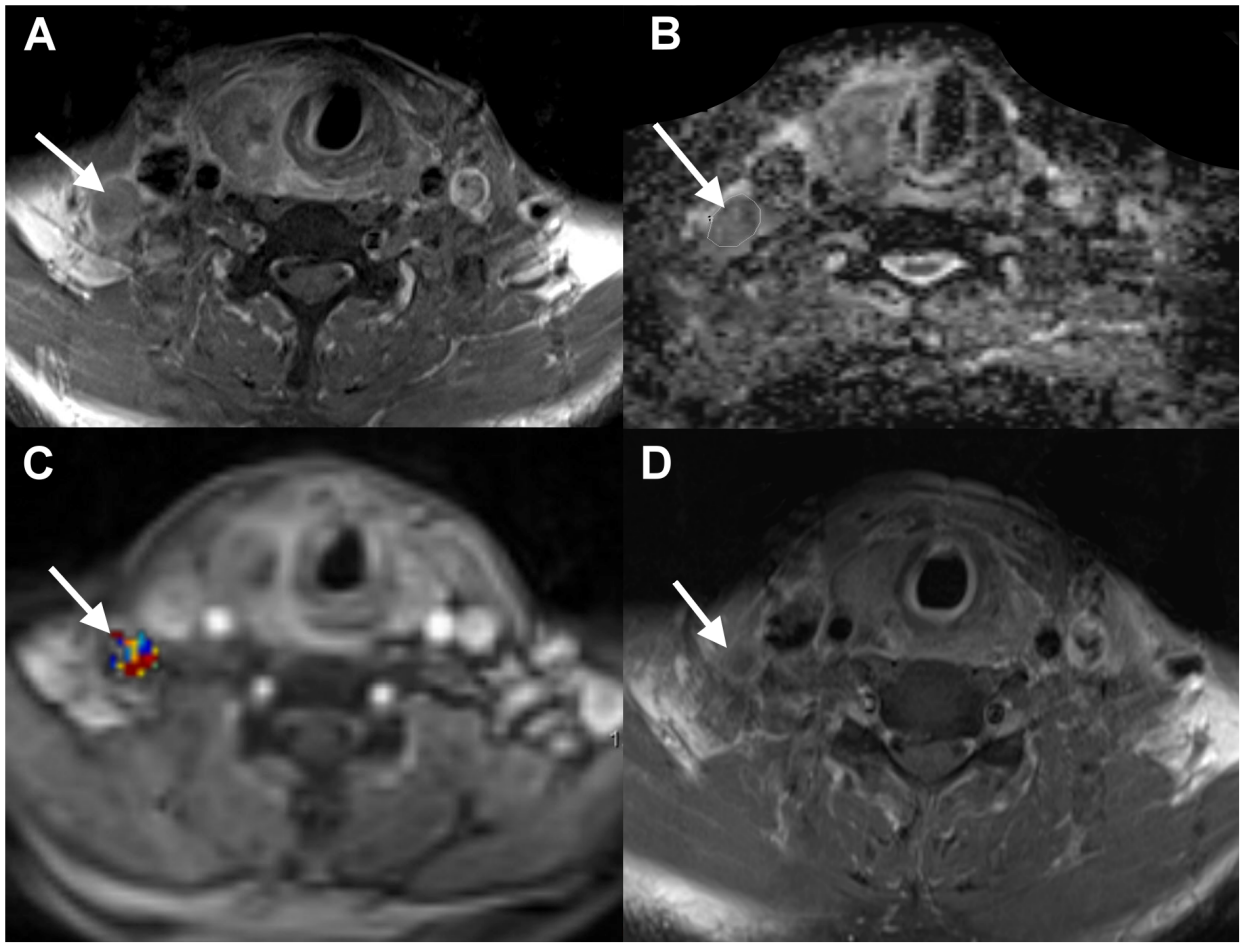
Neck failure in a 45-year-old male patient with right hypopharyngeal SCC after chemoradiation. The patient’s hemoglobin level was 12.7 g/dL. A. Pretreatment axial-enhanced MRI identified a right cervical metastatic node (arrow). B. The corresponding ADC map showed a nodal ADC value of 1.24×10^−3^ mm^2^/s. C. The corresponding PWI map showed a nodal *V*
